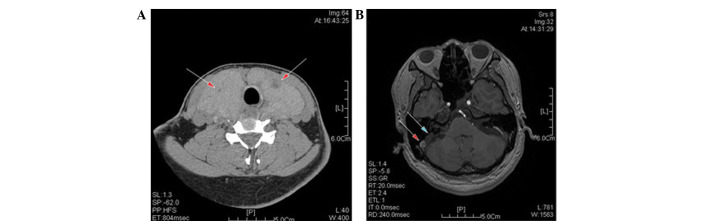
Thyroid ultrasound images. (A) Multiple low echo nodules in each lobe and isthmus of the thyroid (indicated by the arrows). (B) Enlargement of the vestibular aqueducts (indicated by the green arrow) and cavernous sinus (indicated by the red arrow).
